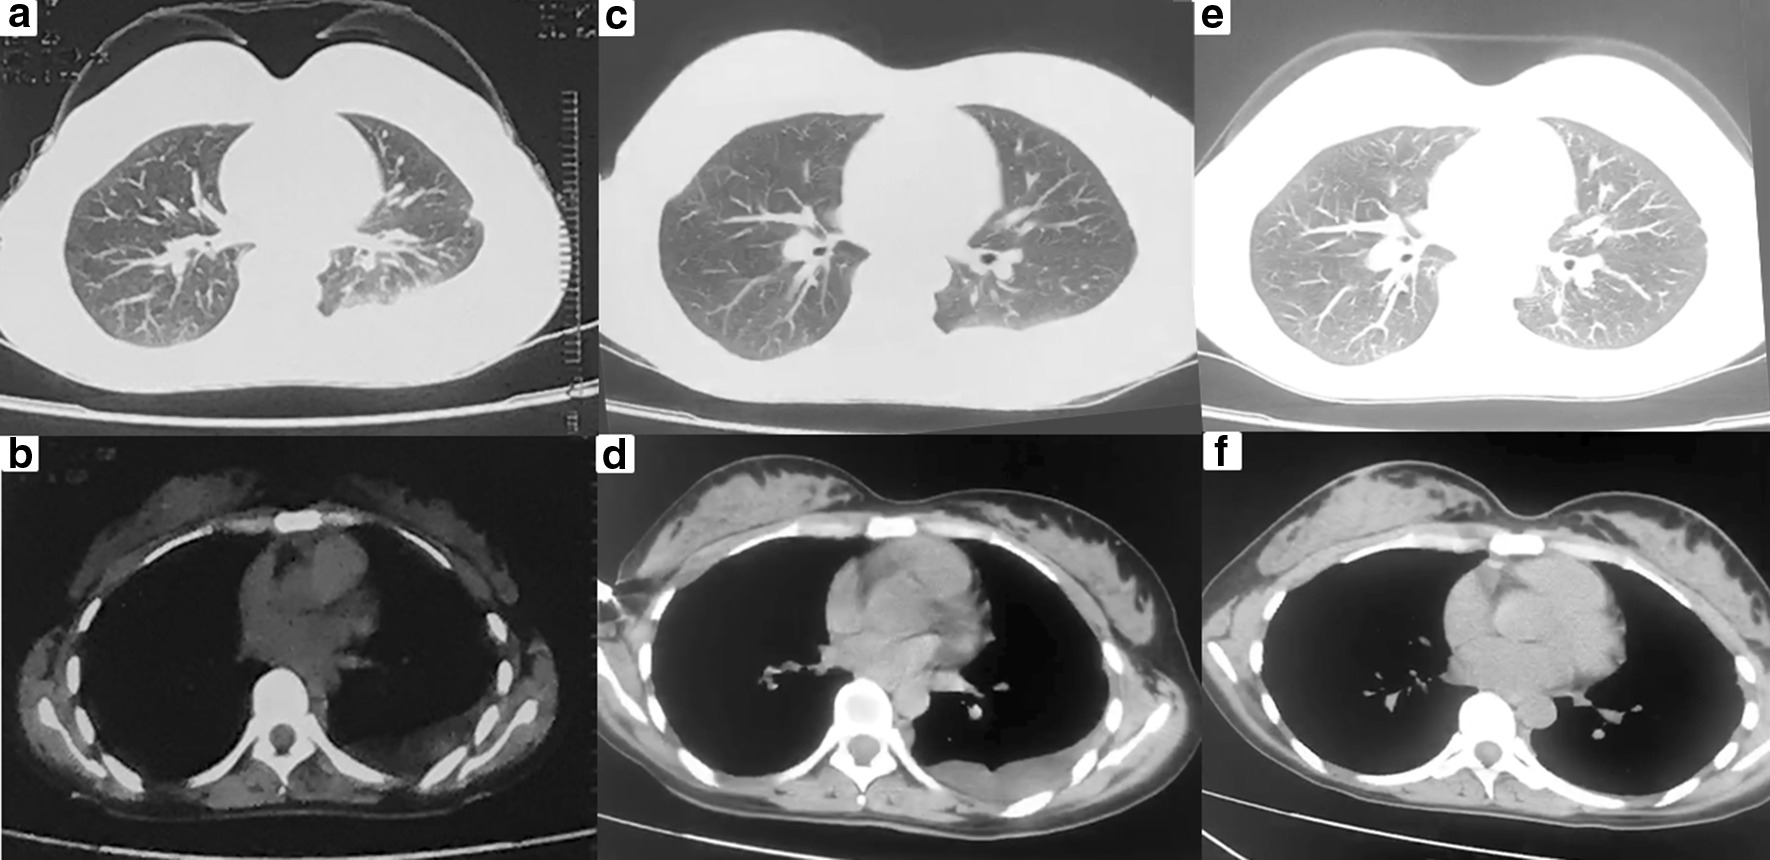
a, b CT scans revealed that left pleural effusion without any nodule in the lung fields. c, d The pleural effusion did not absorb well after 4 weeks of oral corticosteroids. e, f CT scan revealed no pleural effusion after 12 months of oral azathioprine
